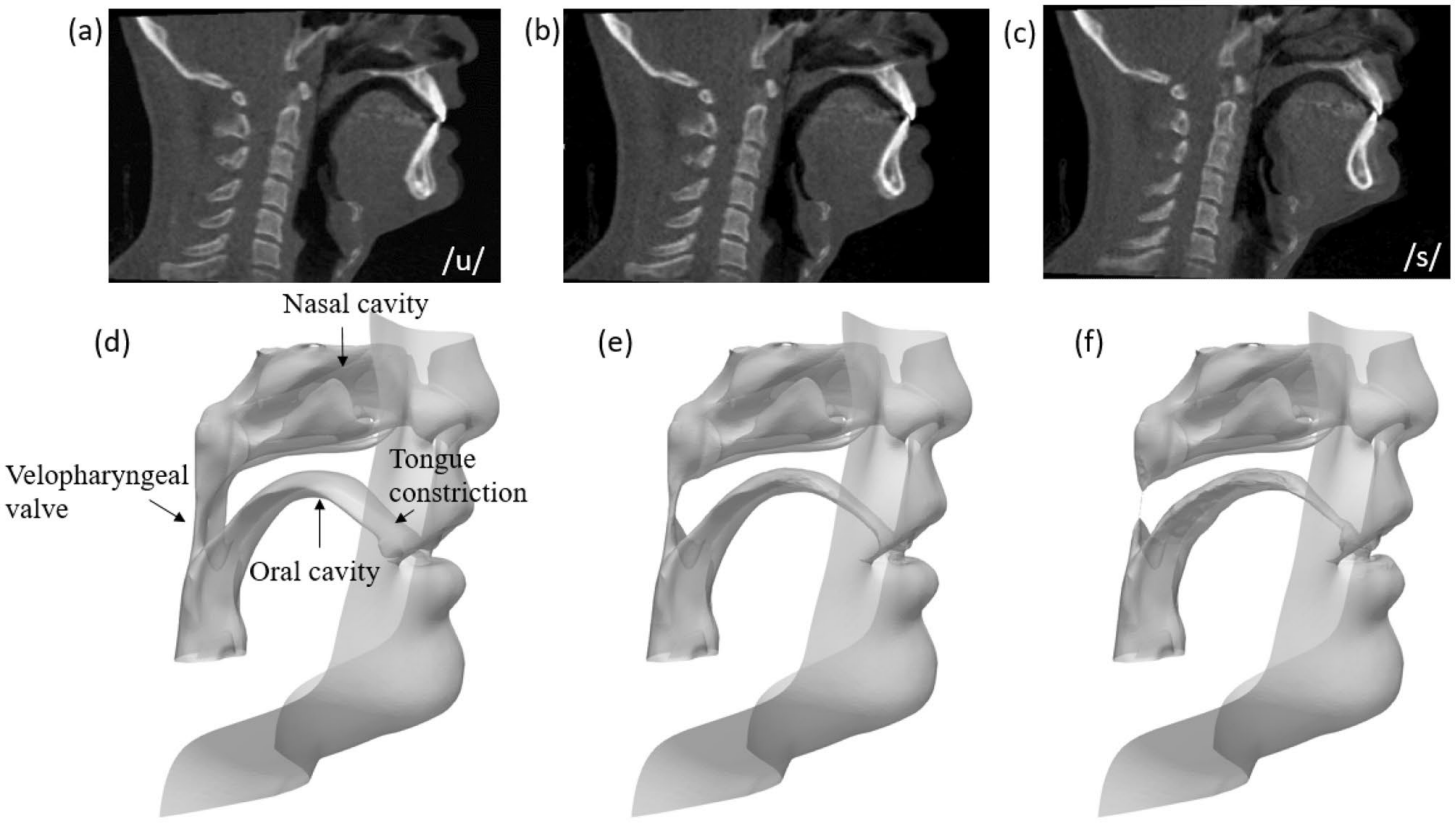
CT images and vocal tract geometry of (a) the end of vowel /u/ to (c) the sibilant sound/s/in the phonation process of /usui/.
